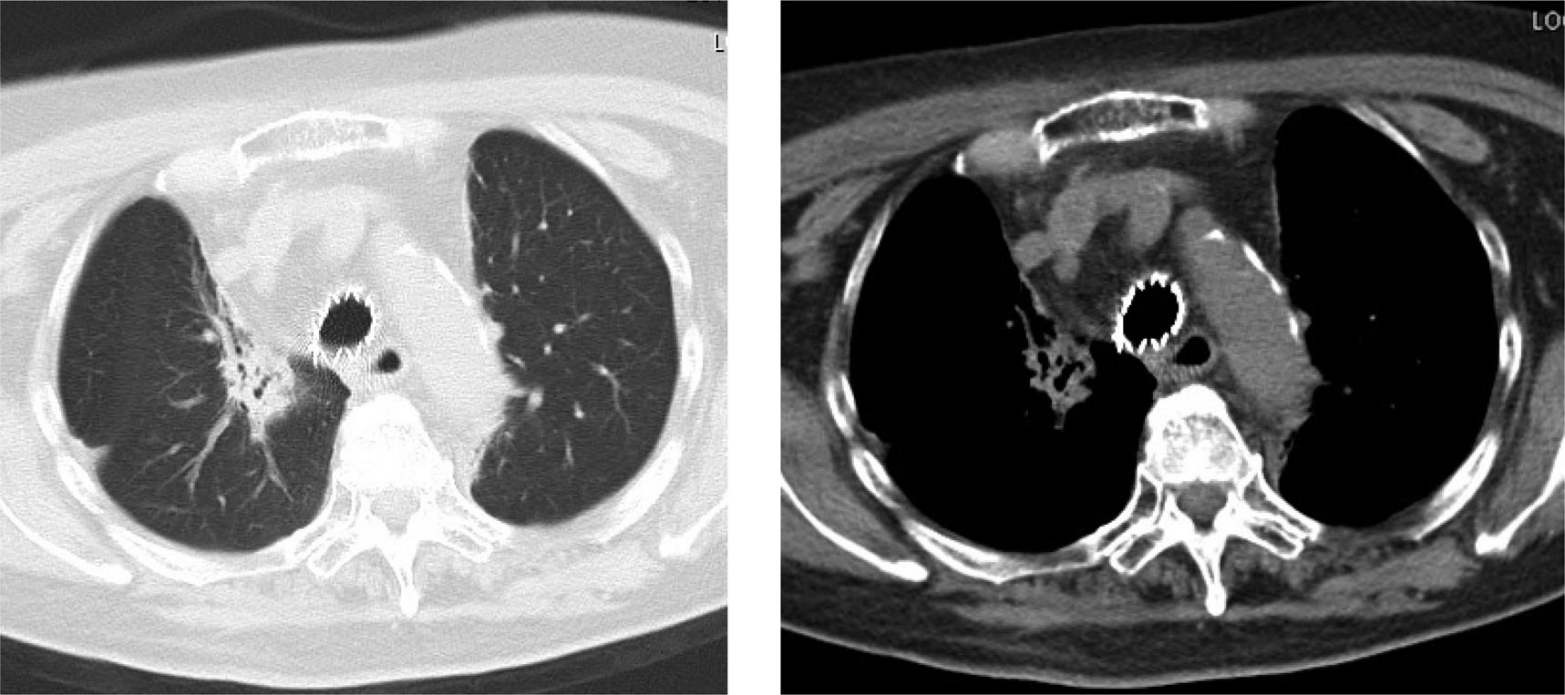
Case 3. RT 60 Gy + ADOC (CDDP+DXR + VCR + CPA) six courses were given. Chest CT five years after stent placement showed good tracheal patency.
